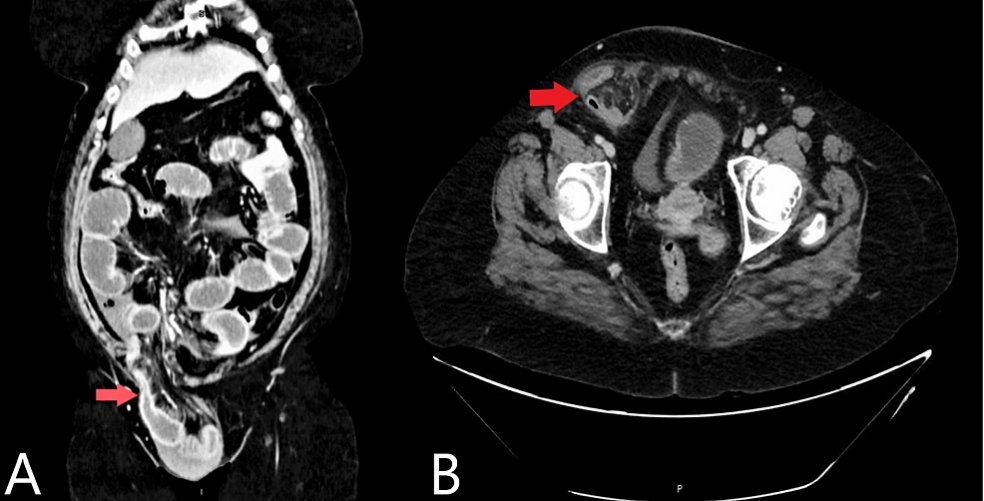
CT of the abdomen and pelvis (A) Coronal view with red arrow to right inguinal hernia. (B) Sagittal view with red arrow to demonstrate right-sided inguinal hernia extending to the right labia suggesting of complicated right inguinal hernia.
